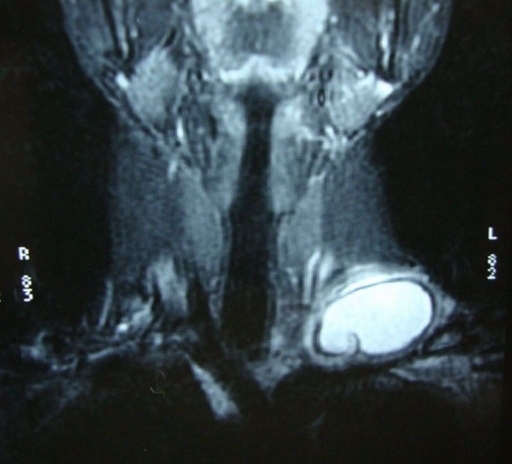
Coronal T2-weighted MRI showing a unilocular, well-defined and high signal intensity cystic lesion with hypointense rim.
